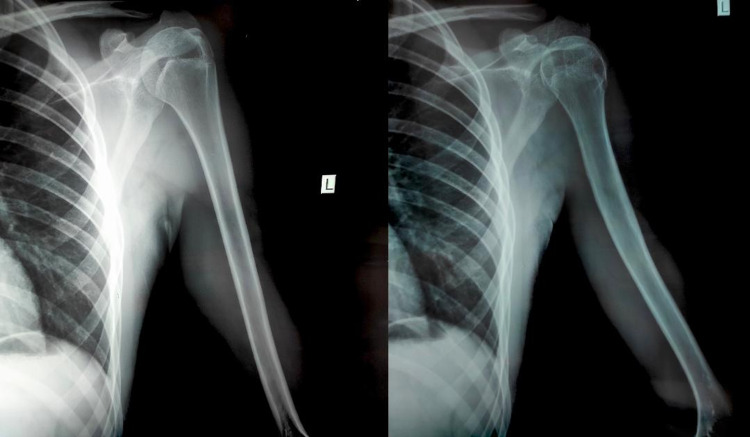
A routine shoulder X-ray due to minor injury shows incidental spontaneous regression of the proximal humerus osteochondroma at a six-year follow-up.
